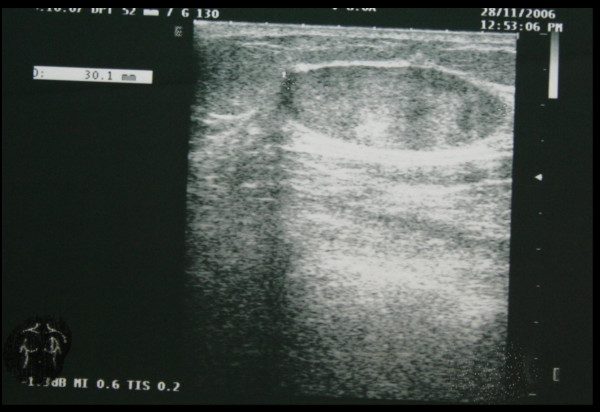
Breast ultrasound showed in the right retroareolar region, a solid mass of 3 × 1 cm with homogeneous echostructure and well-defined margins.
